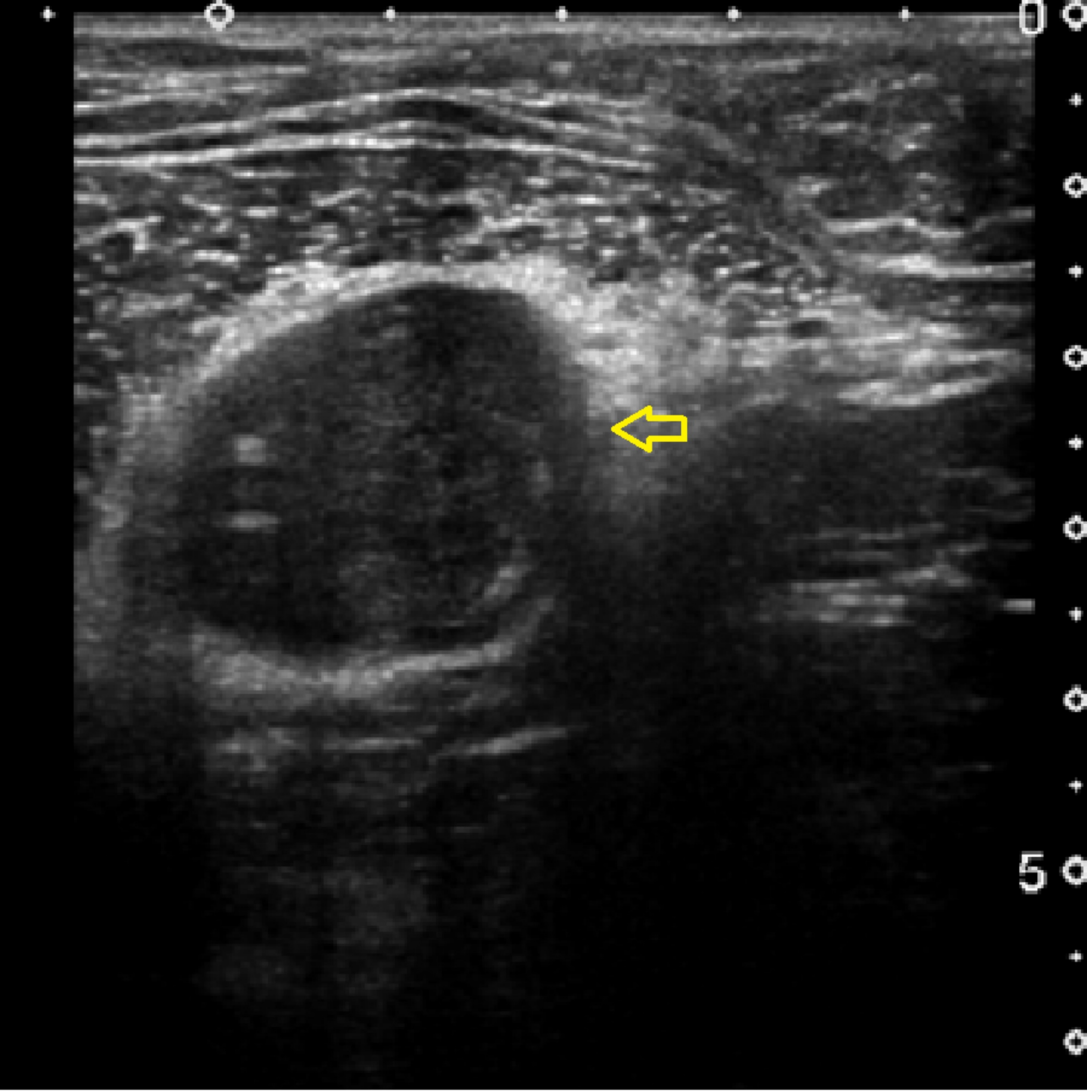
Grayscale ultrasound of chest wall A well-defined hypoechoic solid mass (arrow) noted in between the intermuscular plane showing internal heterogeneity and significant vascularity
